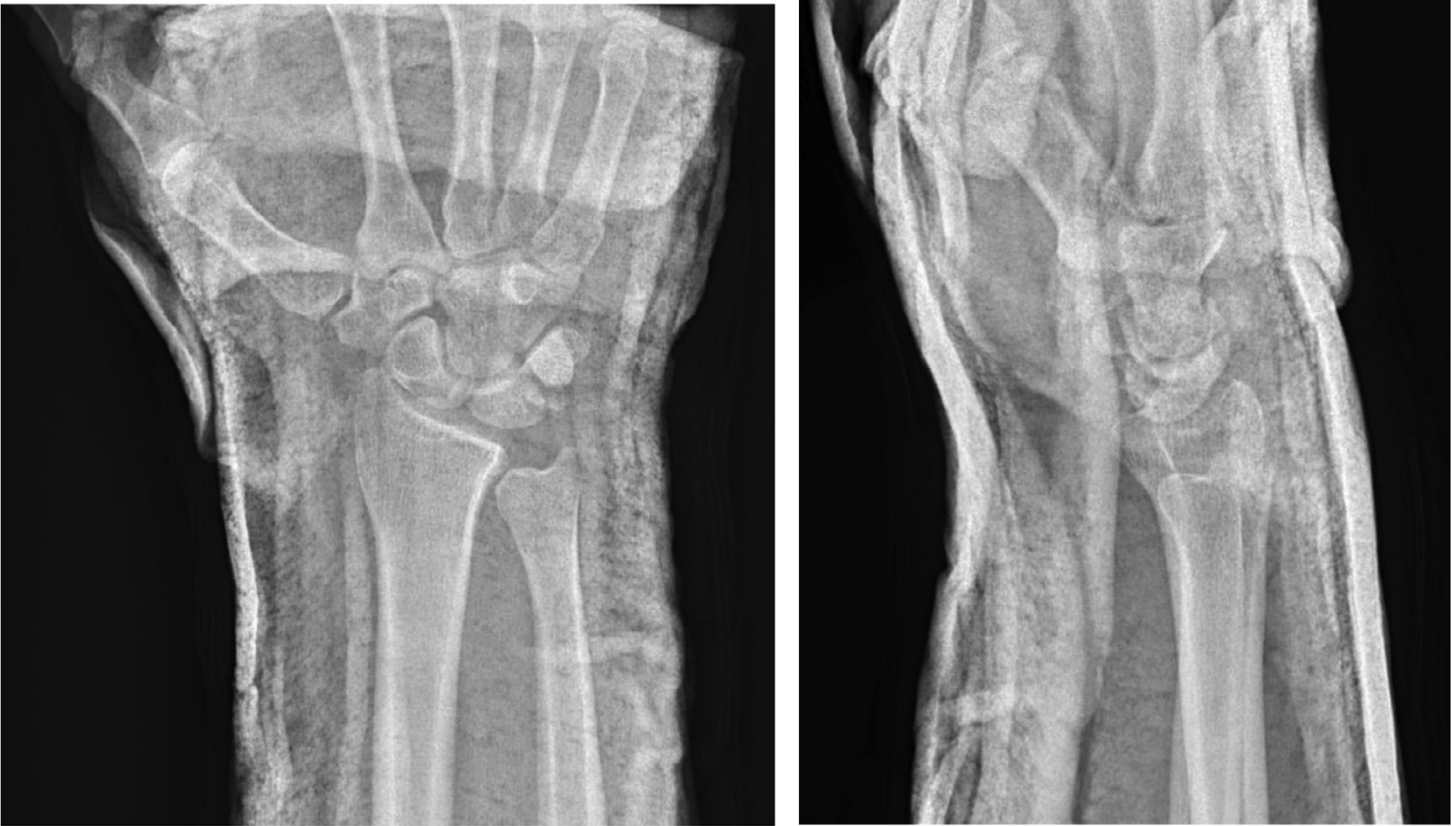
Patient 4: Subsequent radiographs of the right wrist following reduction and splinting demonstrating improved alignment.
